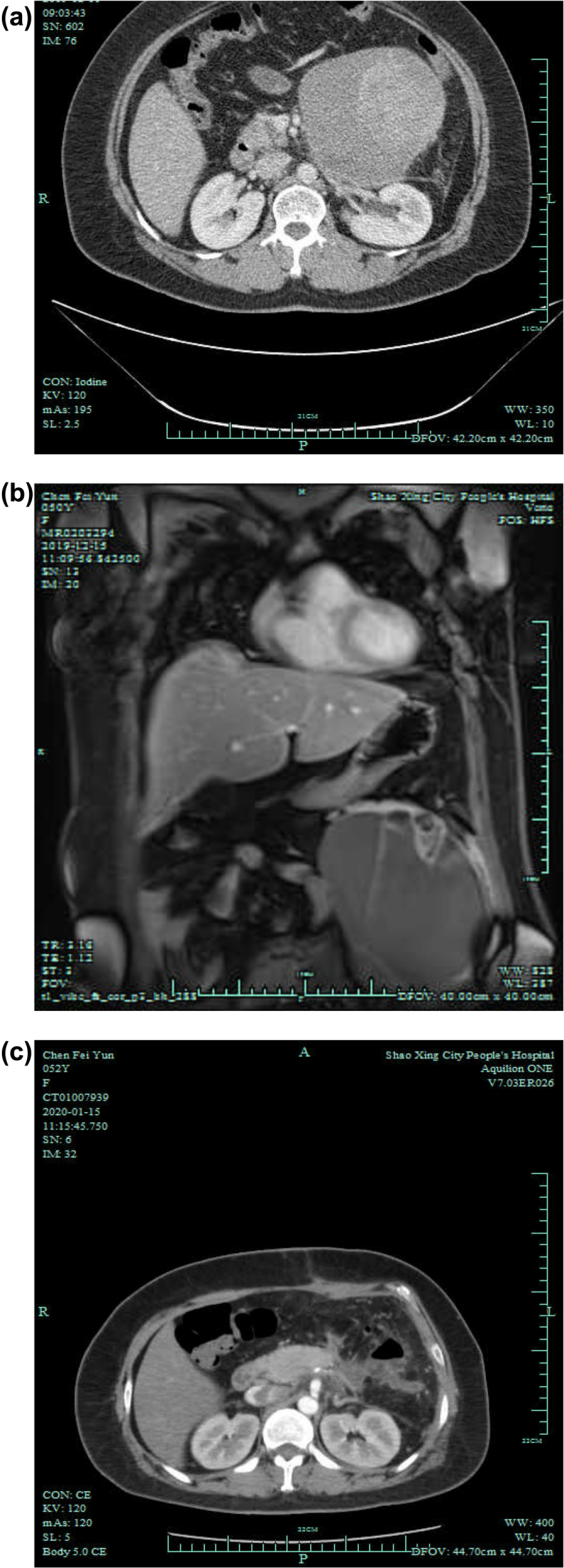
Imaging examinations. (a) Preoperative CT. (b) Preoperative MRI. The left abdominal cavity shows a massive mass (15 cm × 12 cm). There were septa and wall nodules, and the enhanced scanning septum and wall nodules were significantly enhanced. The adjacent peritoneum was slightly thickened, and the adjacent pancreas was pressurized and pushed upward, with an unclear boundary with the lesion. (c) Postoperative CT at 3 months after surgery.
